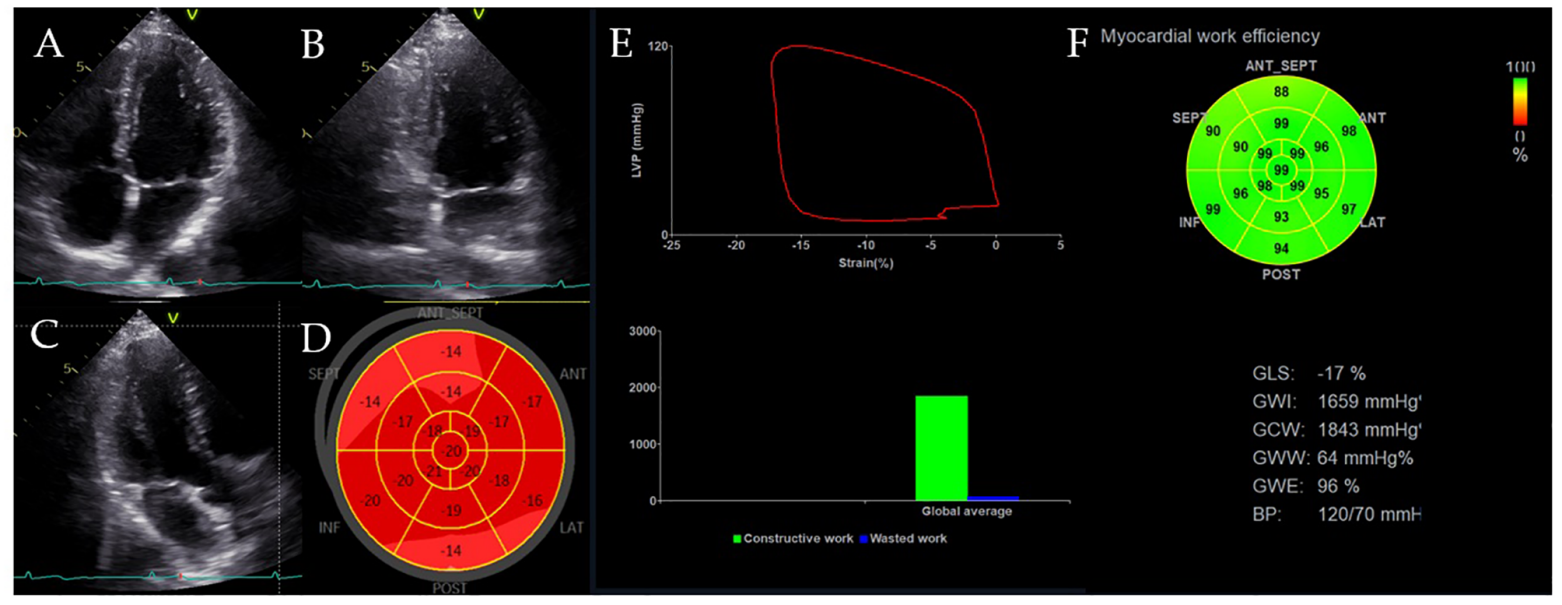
LV pressure-strain loop and MW indices. (A–C) LV-focused apical 4-chamber, 2-chamber and 3-chamber view. (D) Bull’s eye view of LVGLS. (E, F) Pressure-strain loop and global constructive work, global wasted work and global work efficiency.
